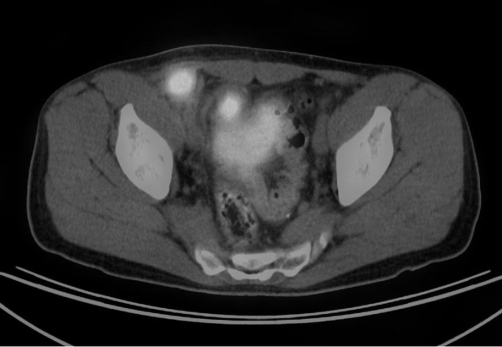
Right lower quadrant mass in PET/CT scan in axial section.
